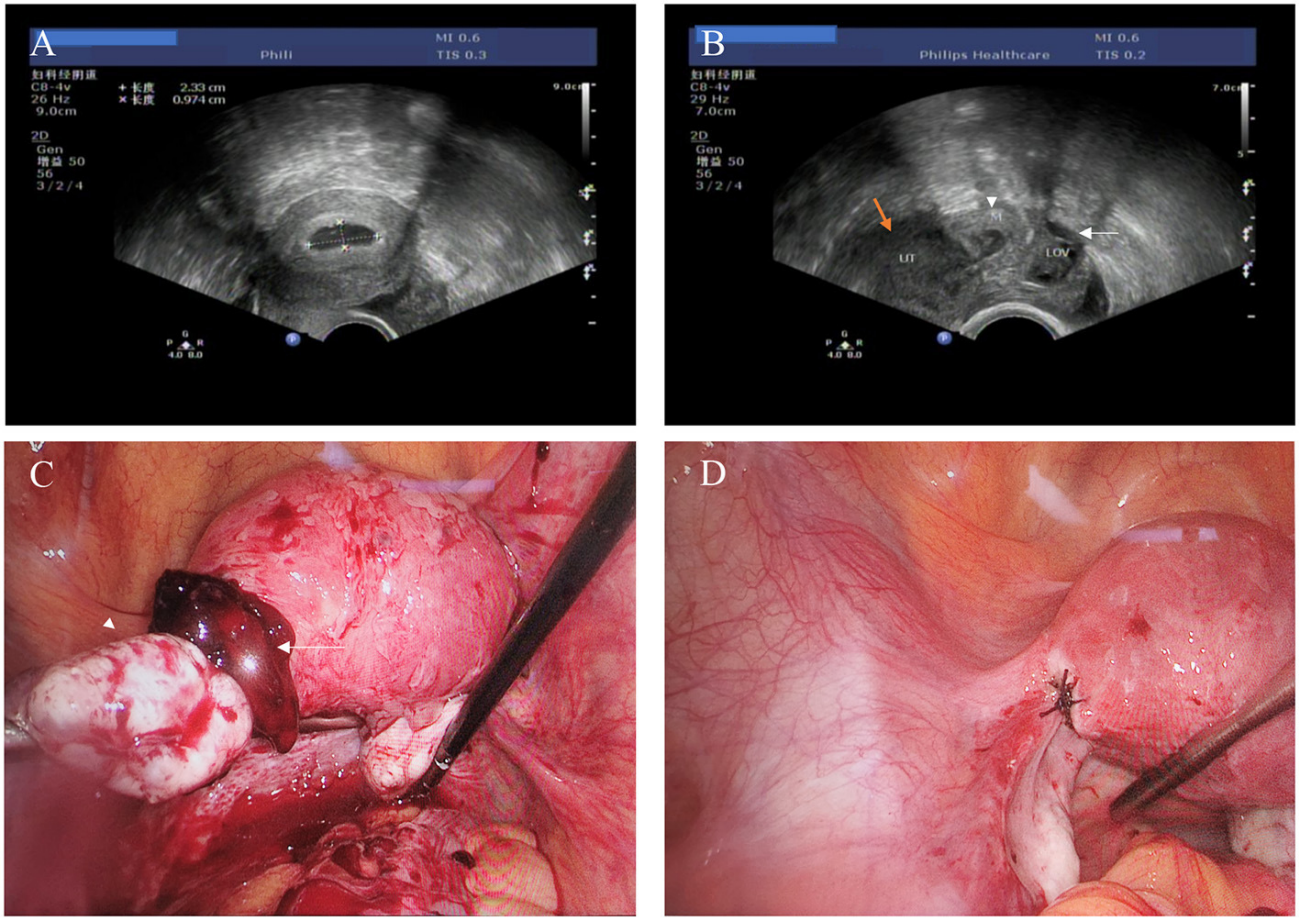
(A) Transvaginal ultrasound examination showing an intrauterine gestational sac. (B) Transvaginal ultrasound examination showing a hypoechoic mass (white arrowhead) between the uterus (orange arrow) and left ovary (white arrow). (C) Laparoscopic findings of an approximately 2-cm blood clot containing chorionic tissues attached to the left ovarian proper ligament. (D) Suture repair of the active bleeding point on the surface of the ligament.
